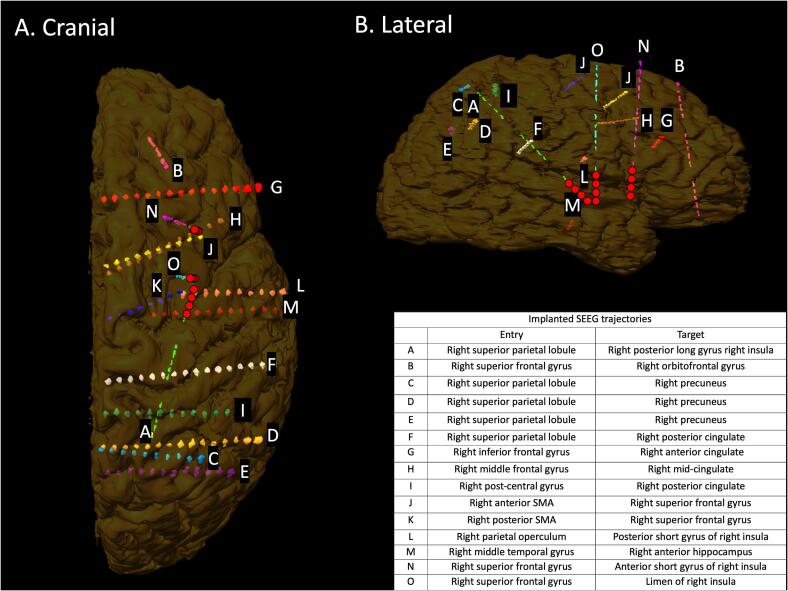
Volumetric MRI registration post-SEEG implantation. Volumetric T1-weighted reconstruction of depth electrodes (A through O) implantation trajectories in the frontal (A) and sagittal (B) planes. Electrode entry points and mesial targets are given in the inset table. Electrodes within the ictal onset zone are marked with red circles. (For interpretation of the references to colour in this figure legend, the reader is referred to the web version of this article.)
